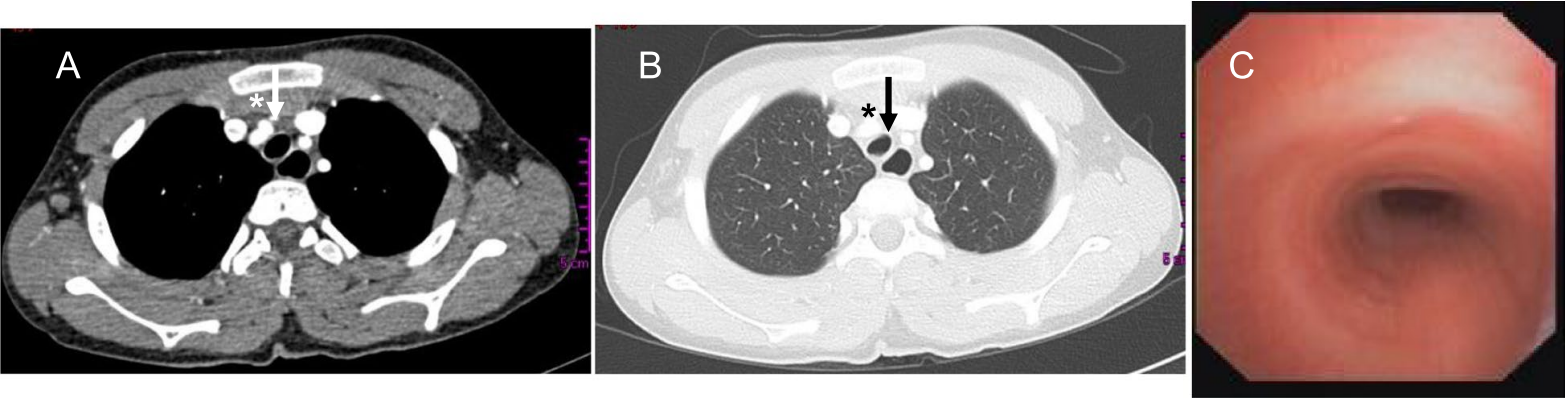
The CT scans from case 1 show (A) the anomalous course of the innominate artery (as indicated by the white arrow and the white asterisk) (B) an anterior compression on the right tracheal wall that induces a reduction of the tracheal caliber (as indicated by the black arrow and the black asterisk) (C) the endoscopic view that confirms the tracheal compression
